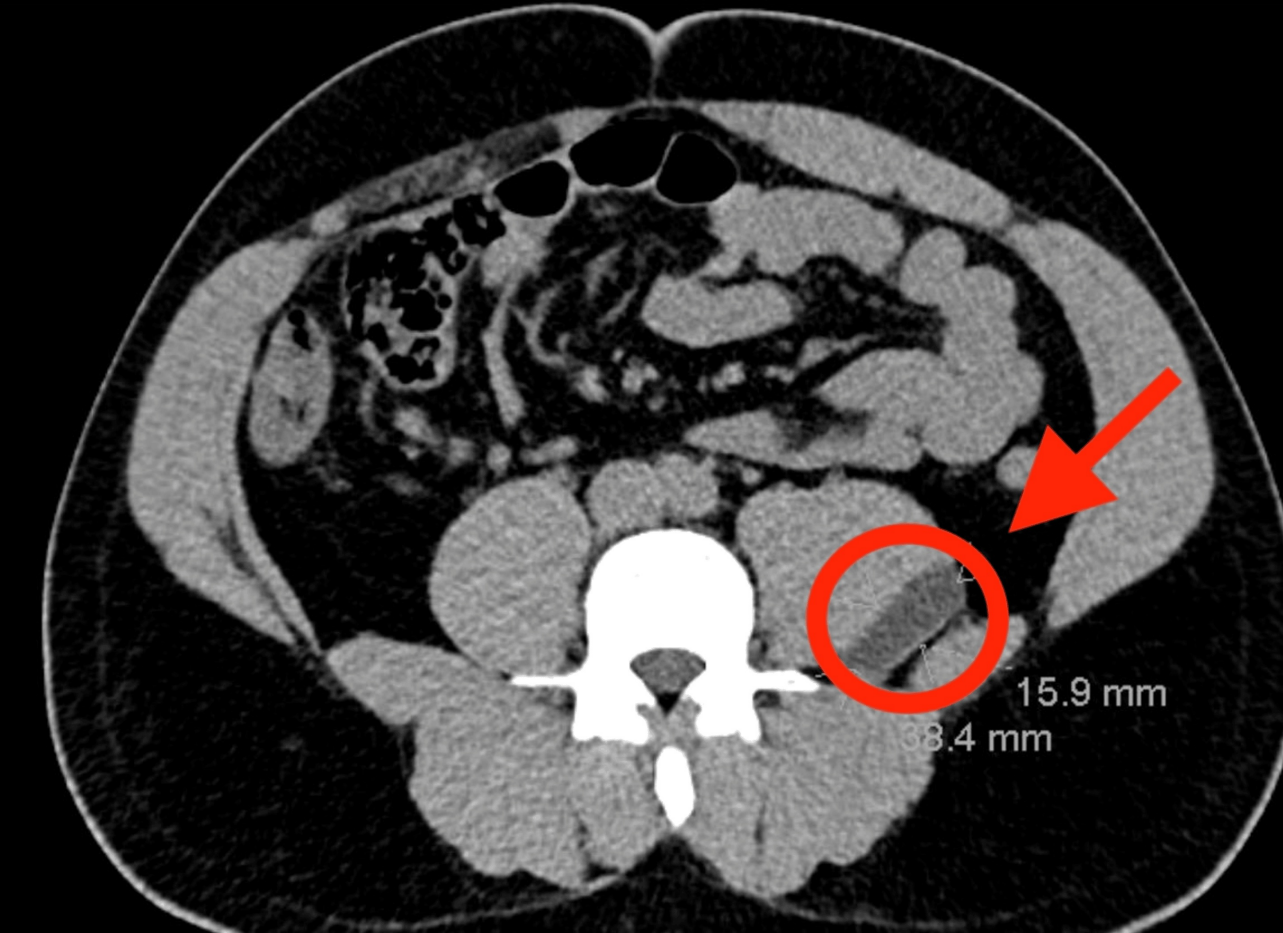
Axial CT of the patient showing psoas abscess (red arrowhead and circle) shown on the posterolateral aspect of the psoas muscle CT: computed tomography
